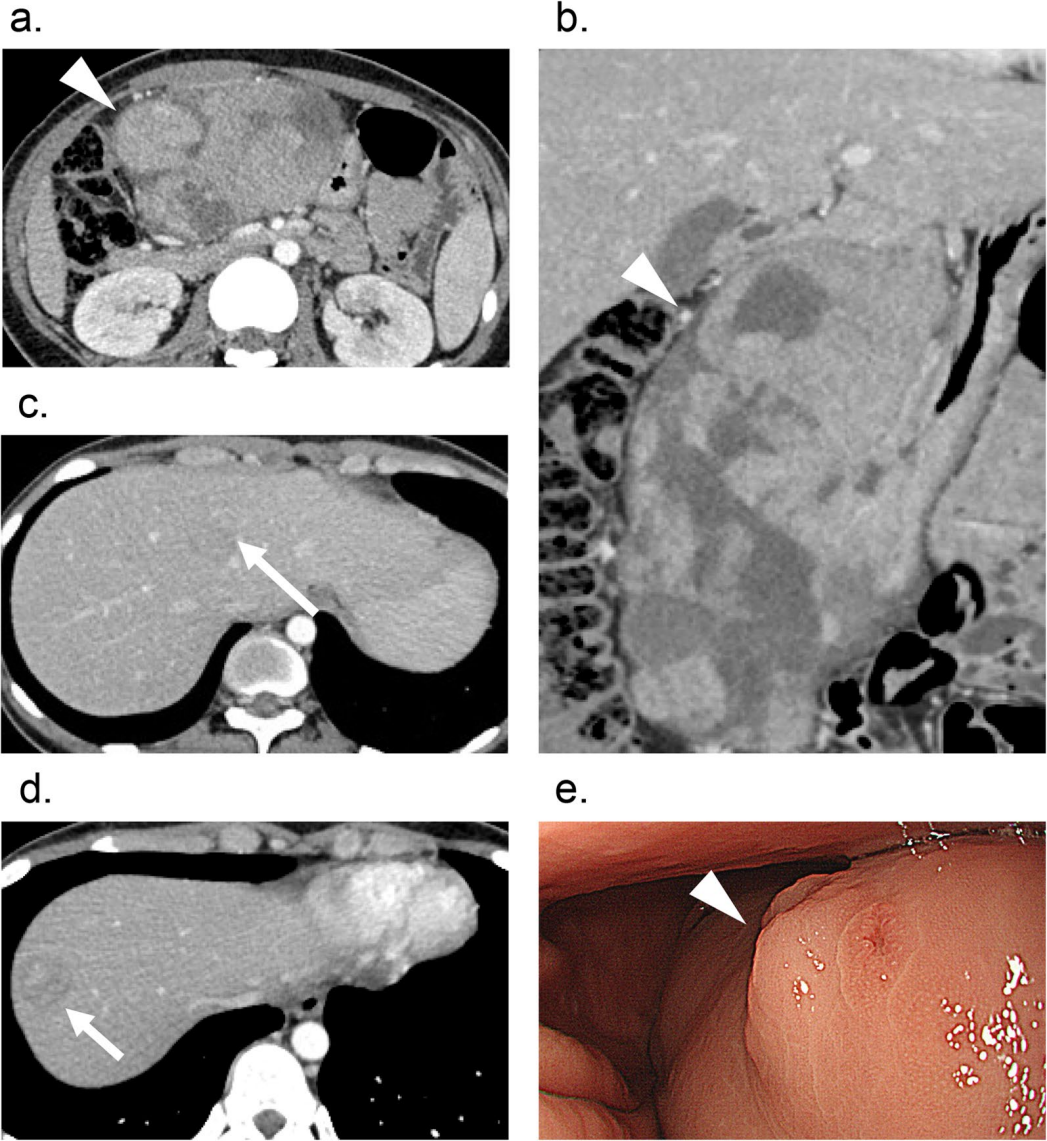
Preoperative imaging findings. a Abdominal/pelvic computed tomography (CT) scan with intravenous contrast shows a huge gastric body-enhancing mass, approximately 17 cm in diameter (arrowhead). b Coronal view of the CT scan demonstrates a huge heterogeneous hypervascular mass (arrowhead). c CT scan showing delayed enhancement of mass lesions in the S4 segment of the liver (large arrow). d CT scan showing delayed enhancement of mass lesions in the S8 segment of the liver (small arrow). e Gastrointestinal endoscopy detected large submucosal lesion > 10 cm in diameter with multiple ulcers on its surface in the lower body of the stomach (arrowhead)
